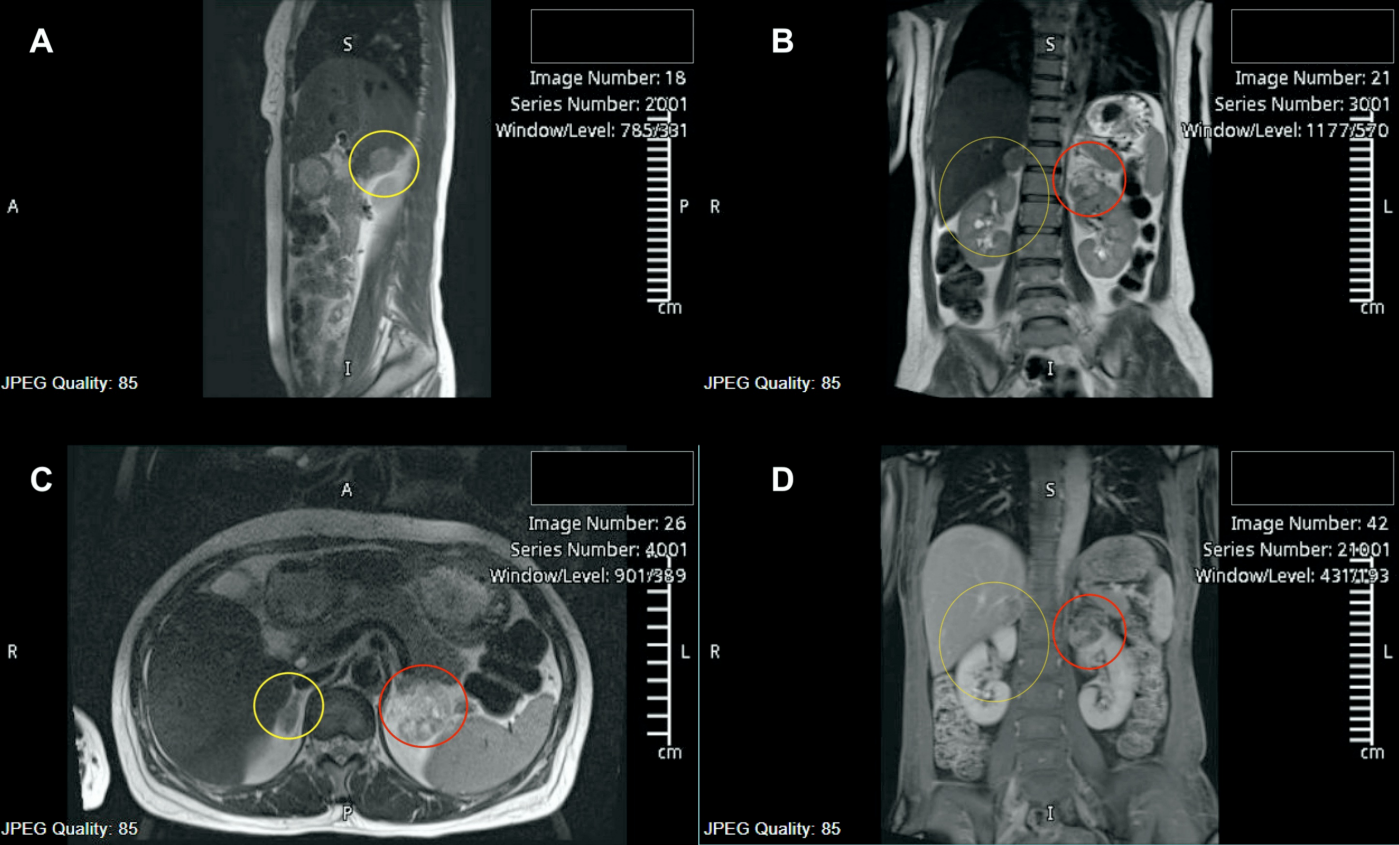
MRI abdomen depicting the right sided adrenal adenoma (circled in yellow), sized 2.5 x 2 cm in different planes. The lesion on the upper pole of the left kidney, sized 3.6 x 3.4 cm (circled in red) was later investigated and turned out to be an angiomyolipoma. (A) Sagittal view, T2 weighted; (B) coronal view, T2 weighted; (C) axial view, T2 weighted; (D) coronal view, T1 weighted.
